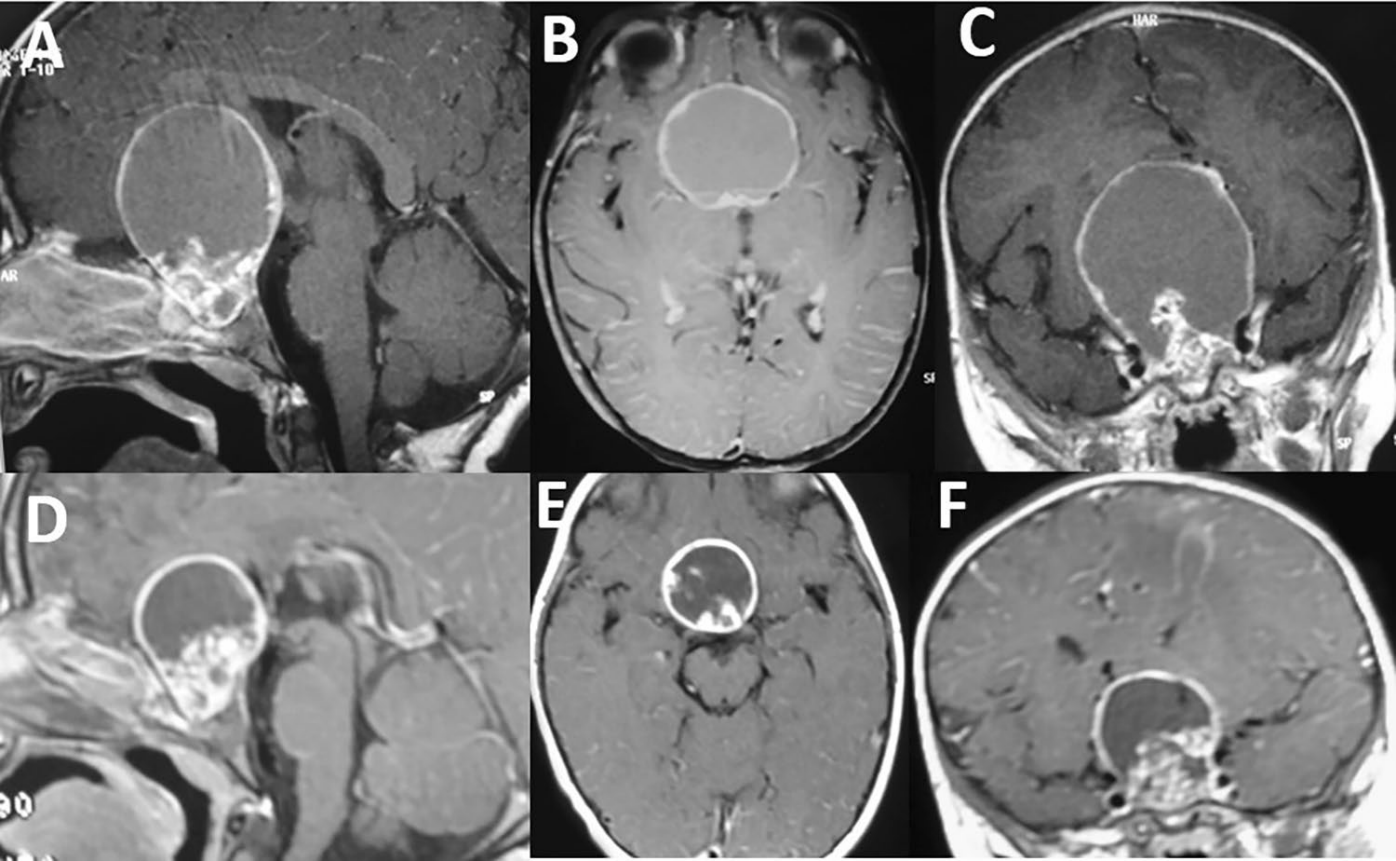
Pre-treatment T1 sagittal (A), axial (B), and coronal MRI (C) of AC in a 5-year-old girl. Note the relatively small, solid, sella portion, and the large suprasellar/third ventricle cystic component. The same sequences performed 2 years later (D, E, F), at the end of 3 cycles of IA, show a quite good control of the disease, with shrinkage of the cyst. The cyst wall remains thick and present a “rigid” appearance
